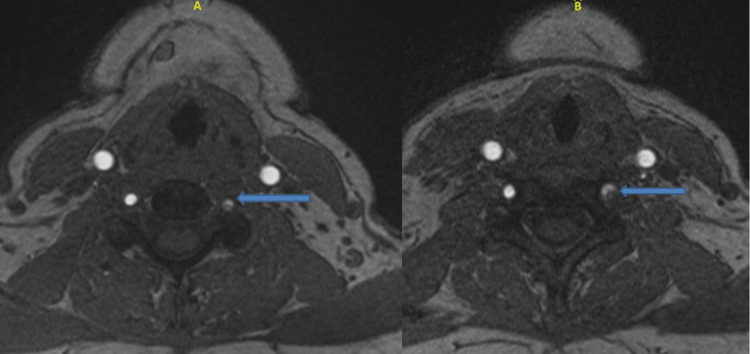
Crescent-shaped high T2 signal intensity within segment 4 of the left vertebral artery Blue arrows: the dissected part of the fourth segment of the left vertebral artery (LVA); picture A: upper cross-section magnetic resonance imaging (MRI) with contrast of the vertebral artery; picture B: lower cross-sectional MRI with contrast of the LVA
